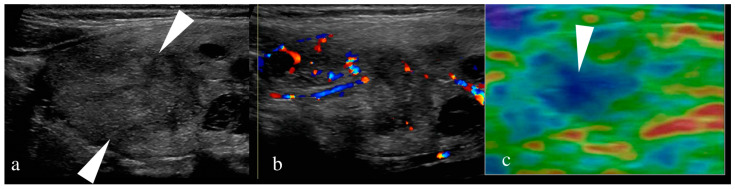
(a–c): (a) Ultrasound of thyroid shows a 3.2 cm solid, hypoechoic nodule (arrowhead), wider than taller with micro-lobulated margin without any micro-calcifications. (b) The nodule shows internal vascularity on color doppler image. (c) Elastography of the nodule shows hard consistency seen as blue colour (arrowhead). In colour elastogram, tissue hardness increases in ascending order from red, yellow, green and blue.
